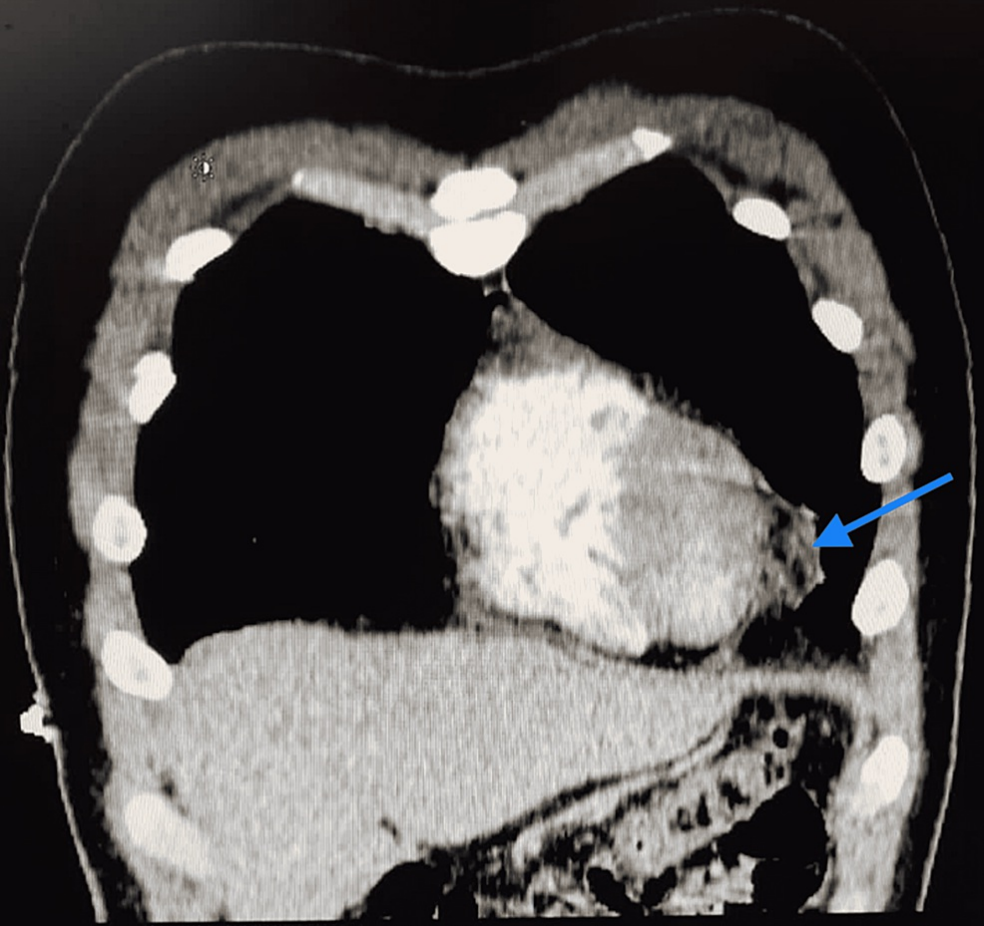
Sagittal view of the pericardial fat stranding (blue Arrow) shown on the CT angio.
